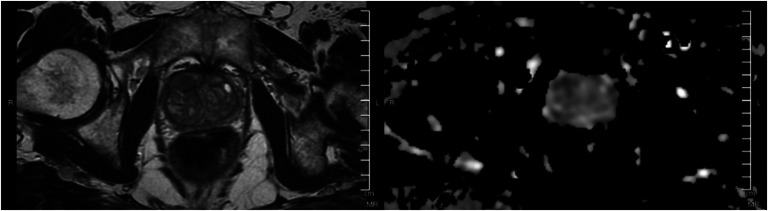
MRI images used for Fusion biopsy. T2W images (left) and ADC images (right) show right anterior lesion. All SBs were negative. 3/3 TBs were positive for Gleason 4 + 3 PCa. This patient went on to have radical radiotherapy. MRI, magnetic resonance imaging; PCa, prostate cancer; SB, systematic biopsy; TB, targeted biopsy.
